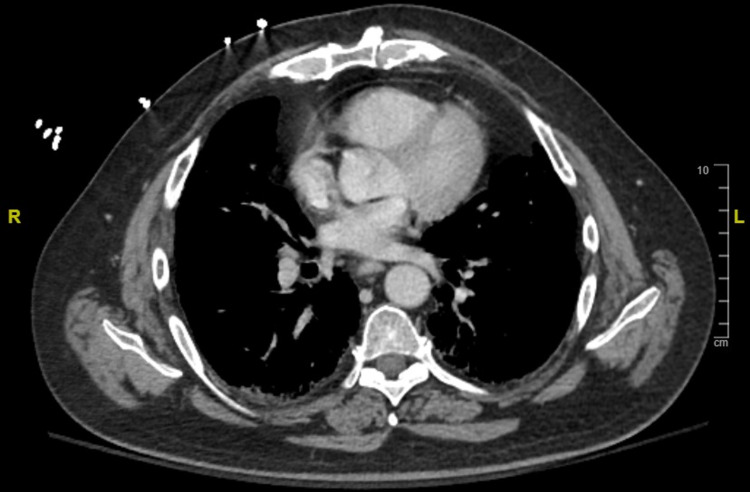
CT of the chest without contrast with no specific findings
